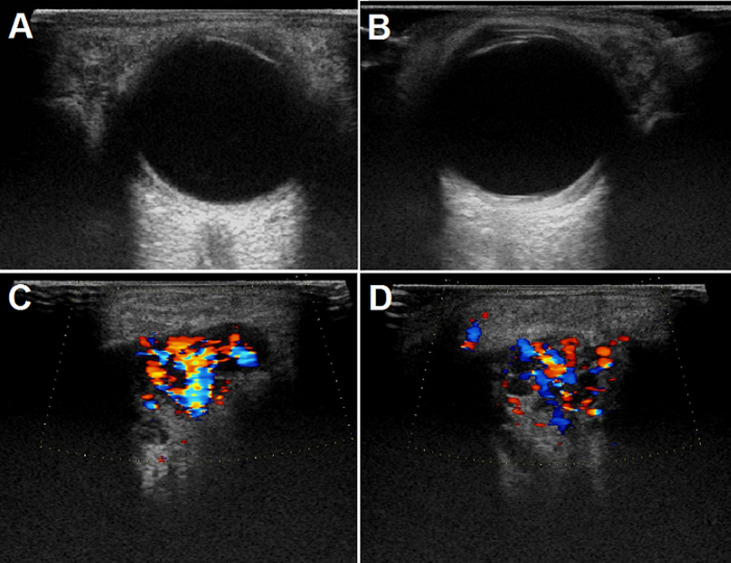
ultrasound of the lacrimal glands before treatment: the left (A) and right (B) lacrimal glands were enlarged (left: 30 x 14 mm and right: 26 x 14 mm) before treatment, with multiple hypoechoic areas; C, D) both glands displayed hypervascularity
